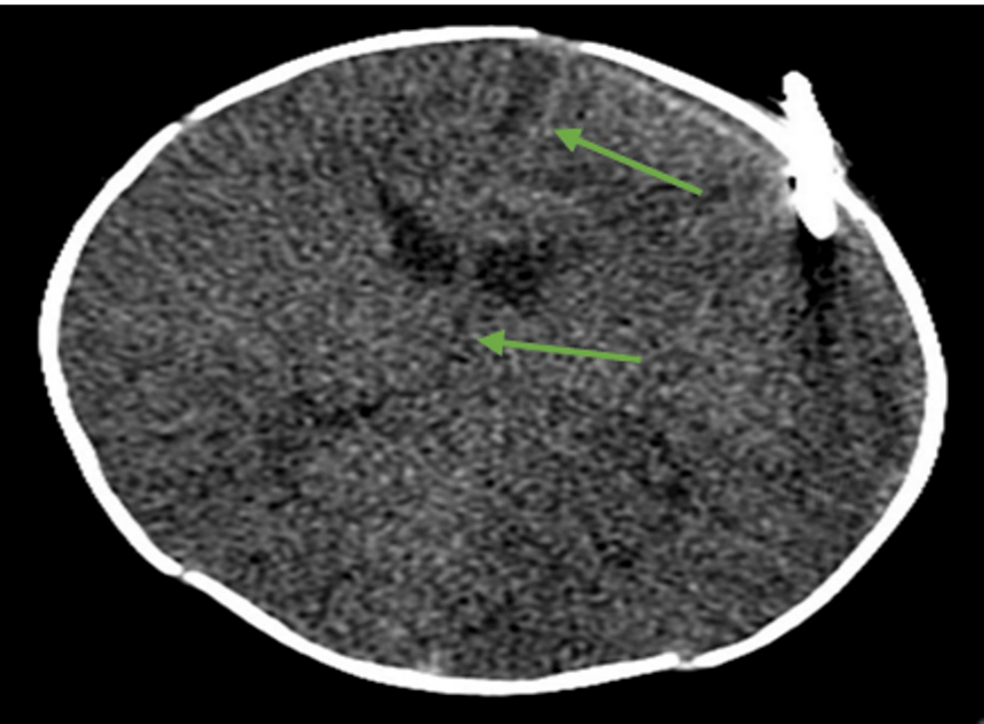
Noncontrast CT brain after interval placement of left-sided subdural drain with significant interval reduction in the volume of the subdural hematoma and significant improvement in mass effect and midline shift measuring approximately 7-8 mm (green arrows).
